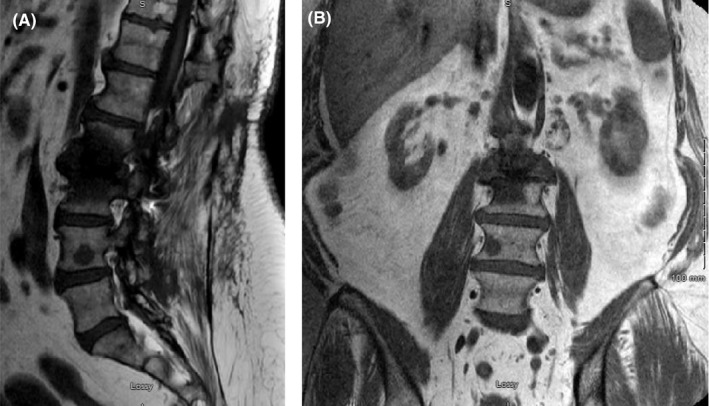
Representative sagittal (A) and coronal (B) MRI sections demonstrating lesions at L2/3 and L4
